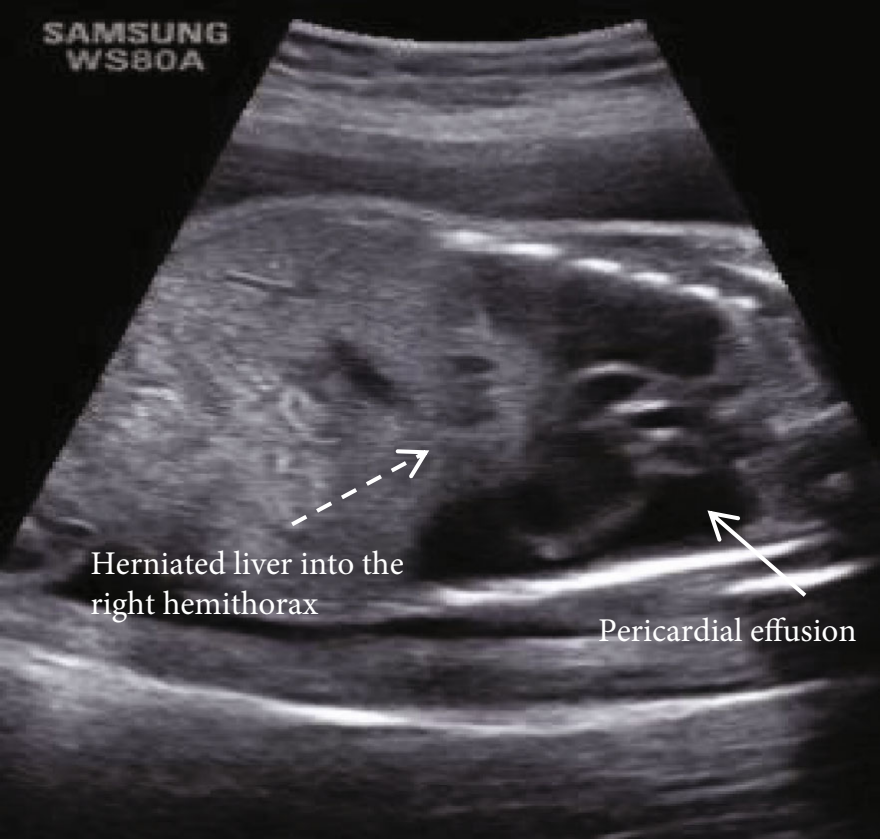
Prenatal ultrasound image of coronal section of the fetal thorax and abdomen, showing a large pericardial collection (full arrow), and an eventrated diaphragm with part of the liver is in the thoracic cage towards the right (interrupted arrow).
